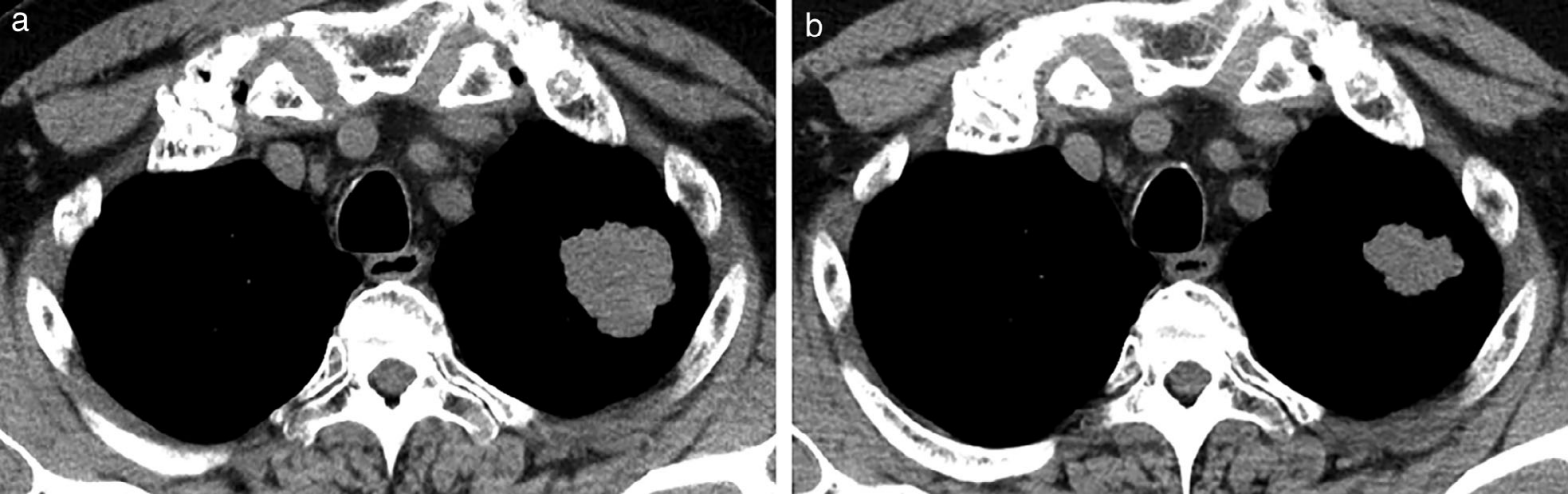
Chest computed tomography in axial slices (a) before; and (b) after three cycles of chemoimmunotherapy induction with atezolizumab, carboplatin, and etoposide. The primary site located at S^1 + 2^a of the left upper lobe shrunk after treatment, exhibiting a partial response in coronal slices (not shown).
